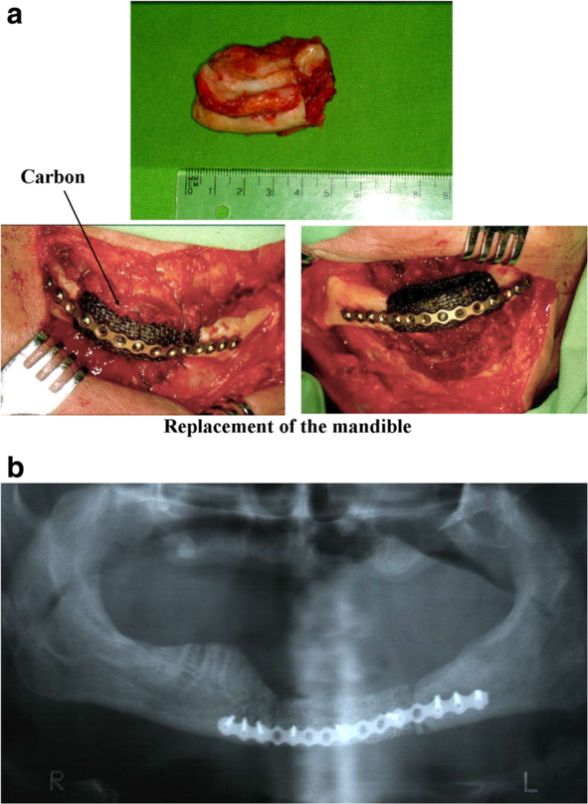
Compact implants with volumes corresponding to the body of the mandible. a Compact Carbulat™ implant, after resection of a gingival carcinoma (post. irrad.). b Panoramic X-ray after the surgery. The density of the Carbulat™ implant is very close to those of human boneA 2-mm-thick net that can be fitted (screwed) to the external surface of the mandible and which follows the curvature of the mandible (Fig. 2).
